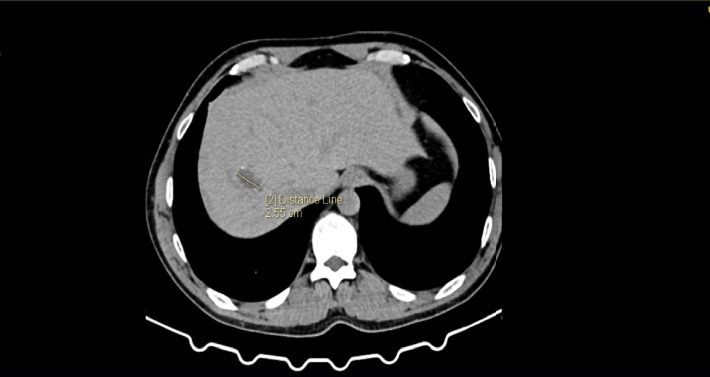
CT scan shows the site of the hepatic echinococcectomy 3 years after the first surgery.
